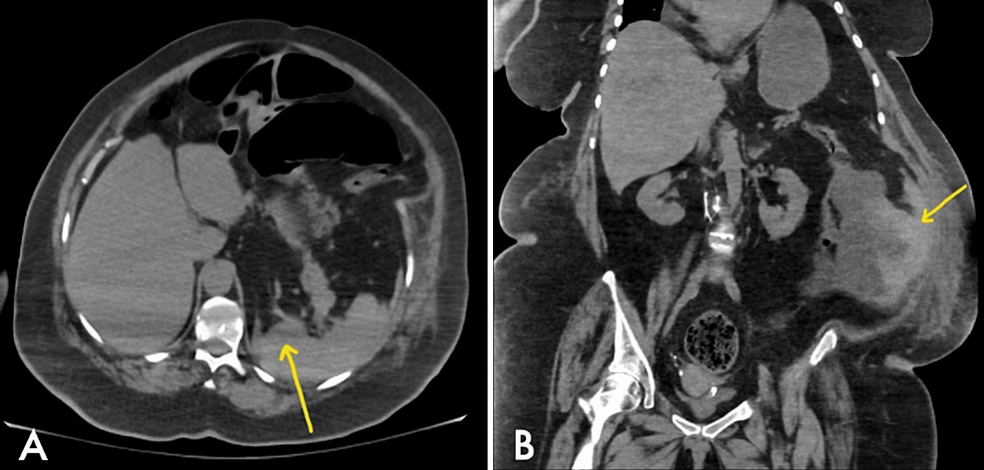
Abdomen/pelvis computed tomography scan: axial view with the yellow arrow showing splenic injury (A), and coronal view with the yellow arrow showing hemoperitoneum (B)
